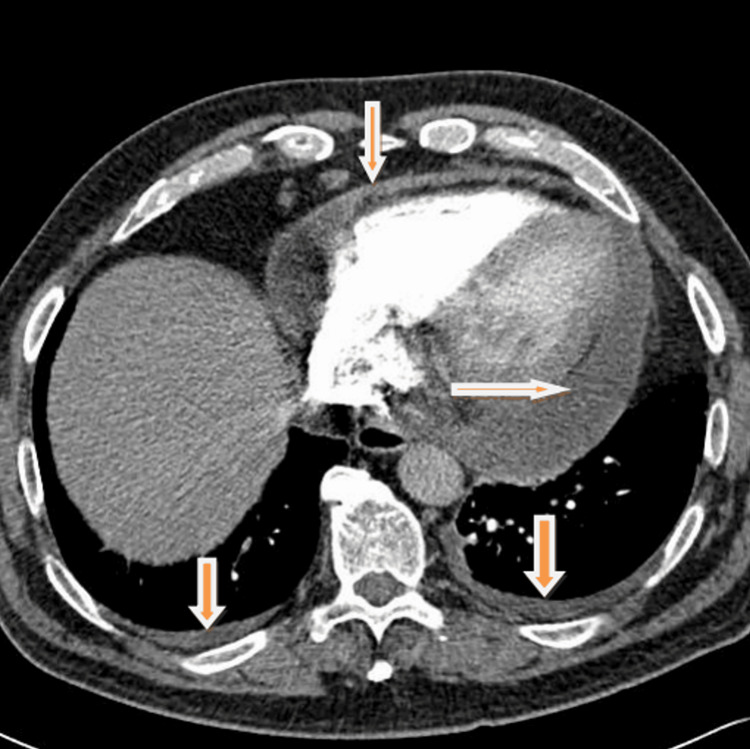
CTPA with pleural effusion and pericardial effusion shown CTPA: computed tomography angiography
